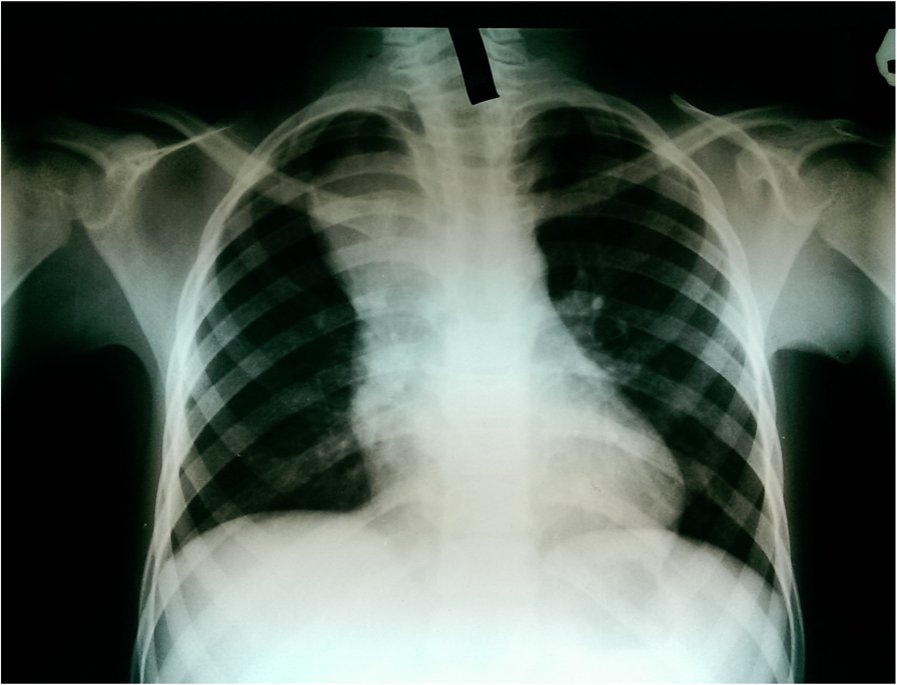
Chest X-ray of the patientᅟ
